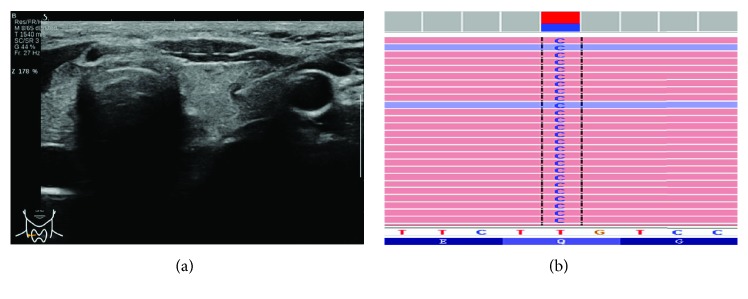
Sonogram of US-guided FNA biopsy for a 33-year-old woman classified as Bethesda III in cytological diagnosis and subsequently pathologically confirmed to have a follicular variant of PTMC (a). NGS of the FNA sample showed an NRAS p.Q61R (c.A182G) mutation (b).
